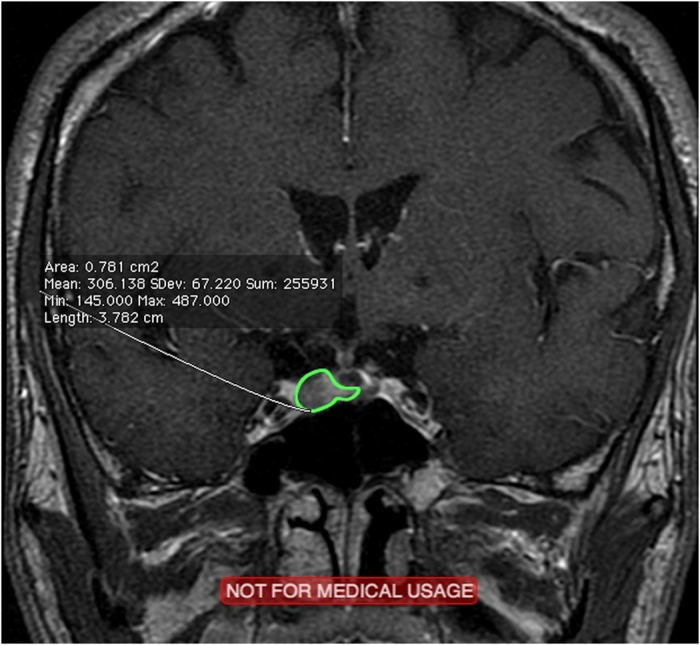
Preoperative MRI of a 57-year-old male patient revealed an extremely small tumor (green area).
